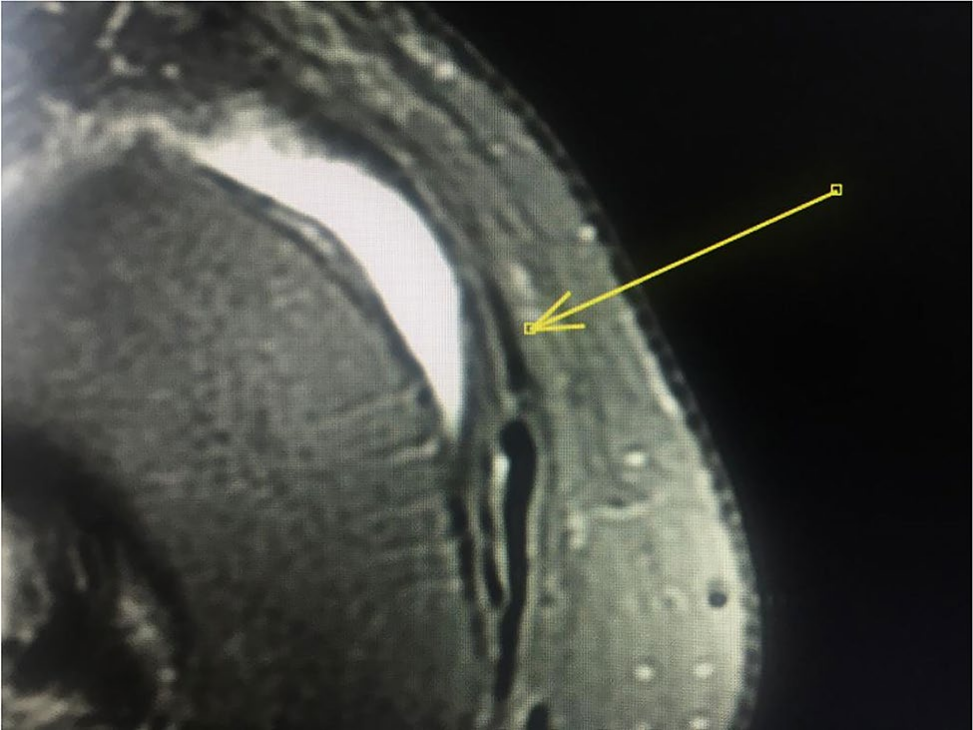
Axial MRI scan showing the AOL(arrow), anterior to the deep and superficial MCL
